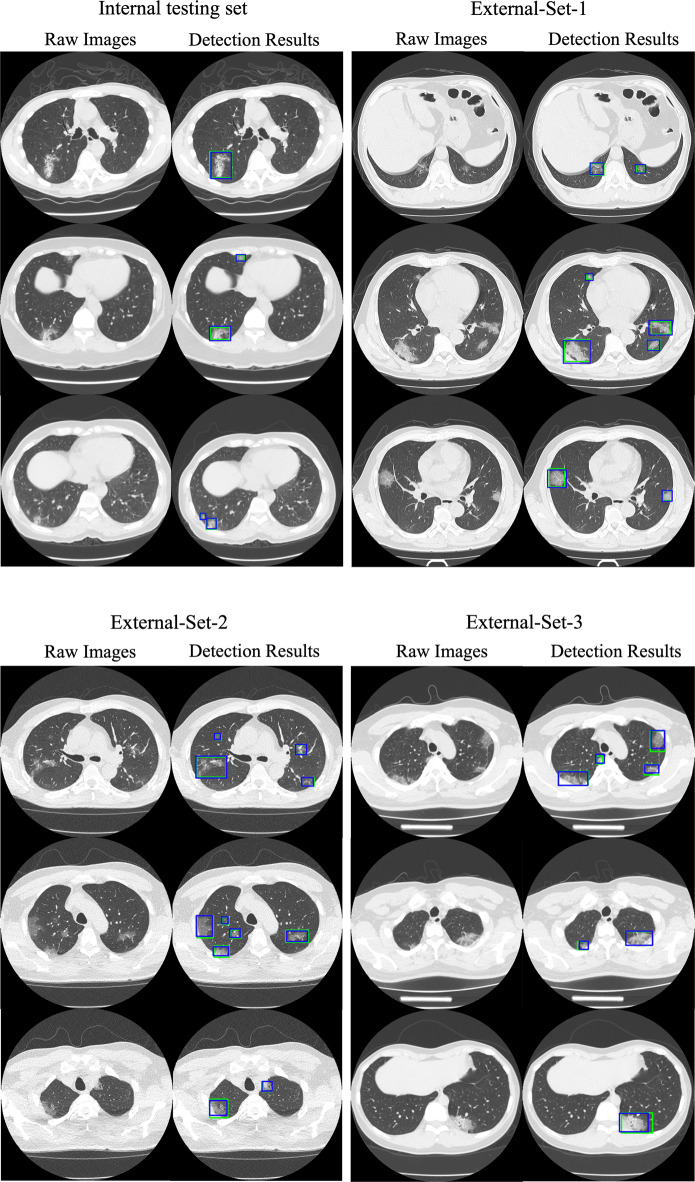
Qualitative results of lesion detection in COVID-19 CT images using federated learning model on the internal testing set and three external sets. The raw images are shown in par with the detection results indicated by bounding boxes. The blue and green boxes denote the model predictions and manual reference lesion regions, respectively.
